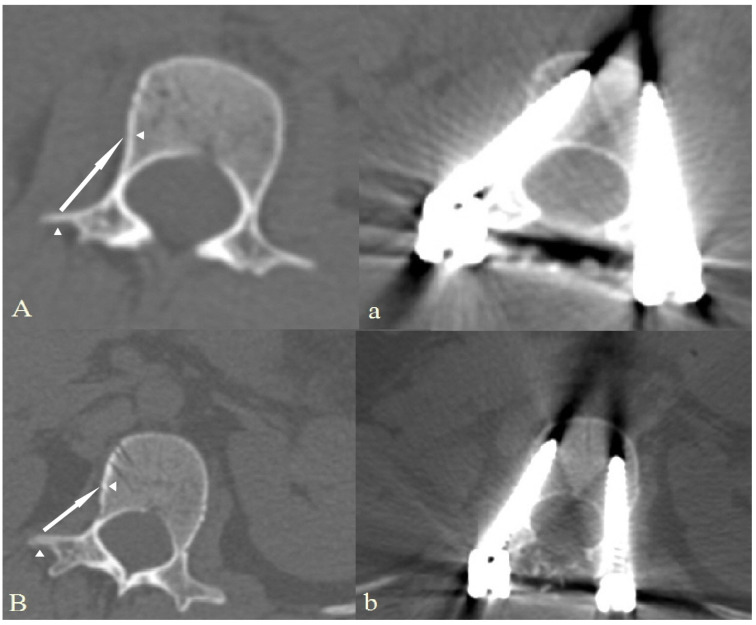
Comparative computed tomography images before (A,B) and after (a,b) screw insertion using our new technique in two patients (A,B). Each angular arrow indicates the cortical bone entry point, and long arrows indicate the trajectory of each screw.
